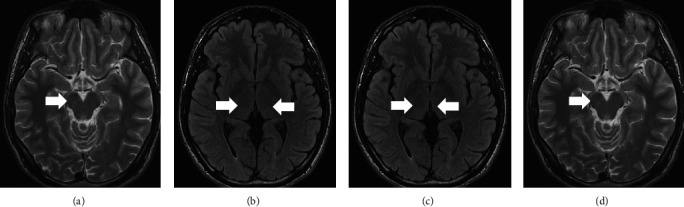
(a) T2WI imaging of the brain 12 days after admission showing the disappearance of the initial lesion (arrow). (b) FLAIR imaging of the brain 12 days after admission showing the disappearance of the initial lesion (arrow). (c) FLAIR imaging of the brain 12 days after admission showing the disappearance of the initial lesion (arrow). (d) FLAIR imaging of the brain 12 days after admission showing the disappearance of the initial lesion (arrow).
